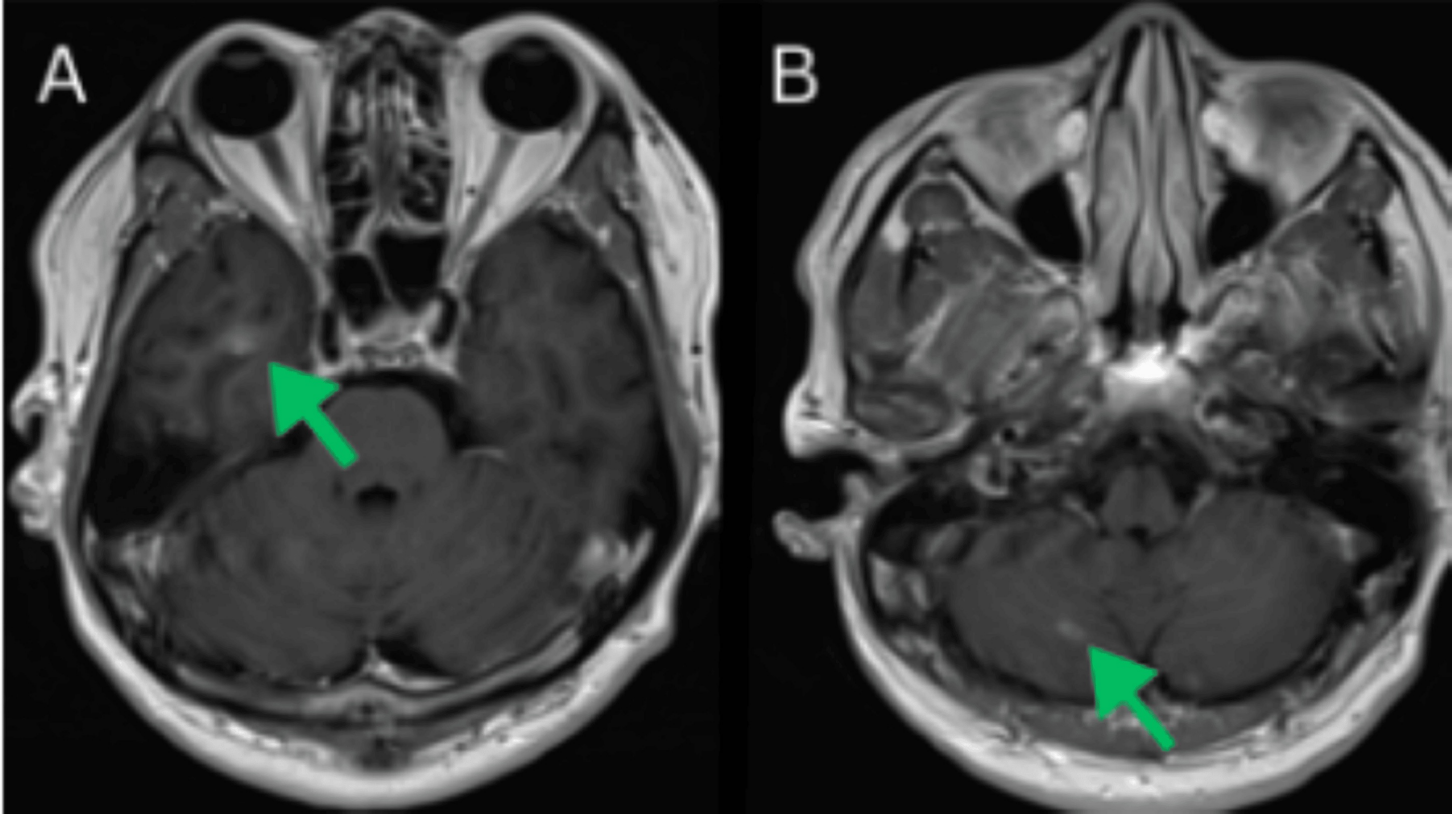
Brain magnetic resonance imaging (MRI) performed at the two-month follow-up A partial radiologic response was observed, with a reduction in the size and enhancement of the lesion in the right inferior temporal lobe (A) as well as the lesion in the right inferomedial cerebellum (B), indicating interval improvement in both regions.
